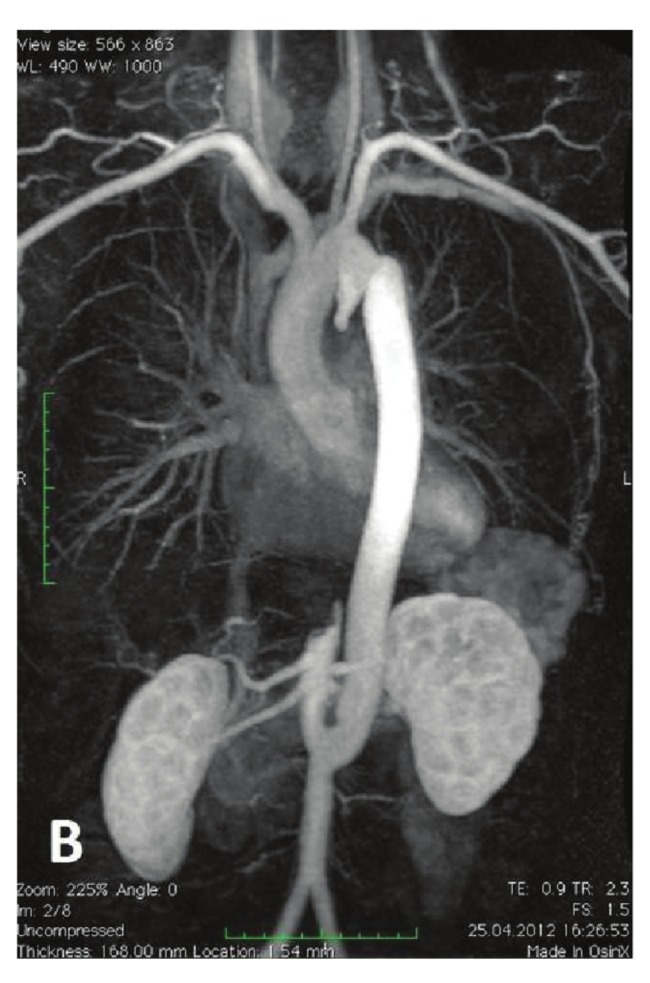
Postoperative MRI of the aorto-aortic bypass showing a patent graft accompanied by a completely occluded native descending aorta.
